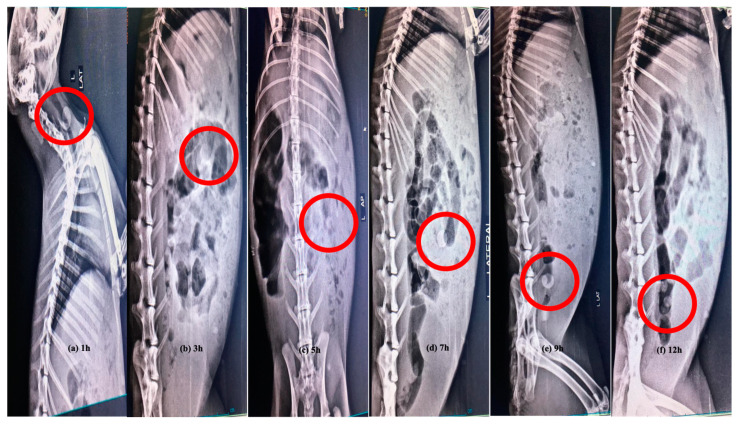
In vivo real-time X-ray images of a hydrogel disc in different segments of the GI tract at different time intervals (a) 1 h, (b) 3 h, (c) 5 h, (d) 7 h, (e) 9 h and (f) 12 h. Red circles specify the position of hydrogel disc.
